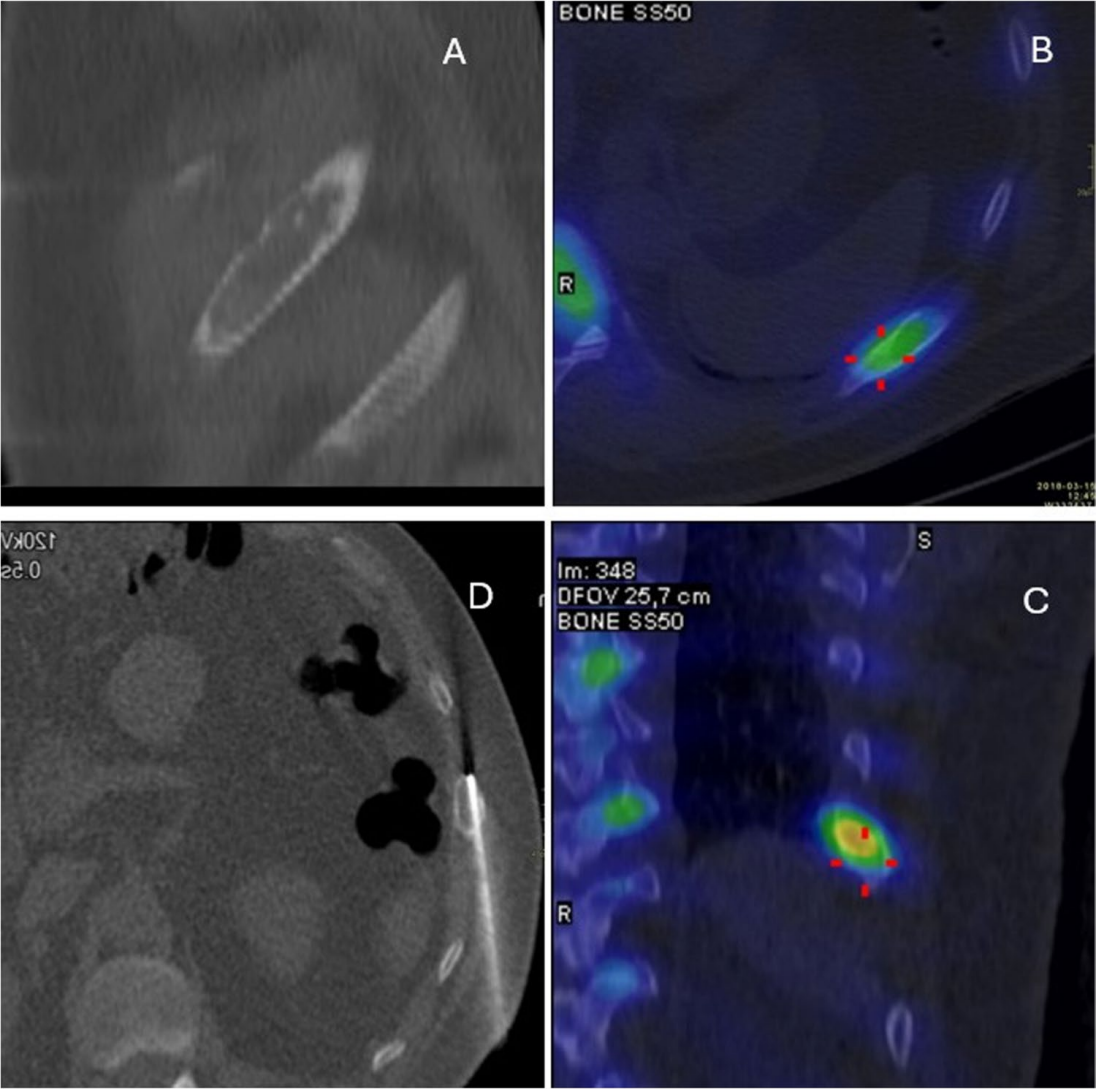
A 65-year-old patient with chronic chest pain. CT shows destruction in the left 9th rib and SPECT-CT shows high uptake in the same rib. Bone core biopsy shows fibrous dysplasia
